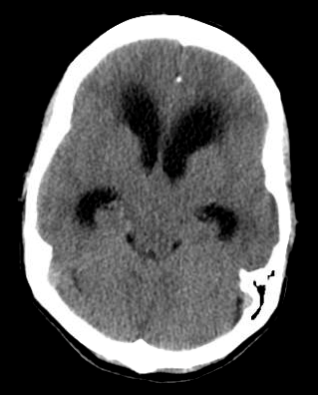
CT of the Brain without Contrast B: Axial image demonstrating possible obstructive pathology at the level of the Foramen of Monro
